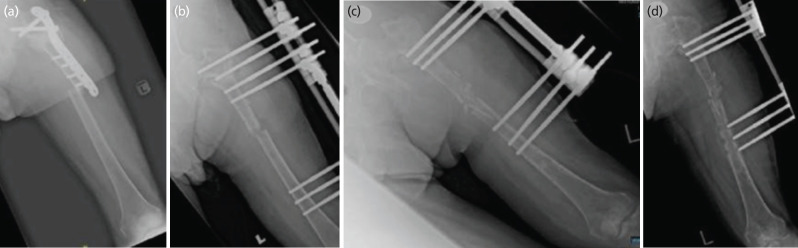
AP radiograph of Left femur showing; (a) peri-implant fracture following fall, (b) post fracture stabilisation with monolateral fixation system, (c) post fracture augmentation with non-vascularised fibula graft, (d) loosening of LRS distal pins.
